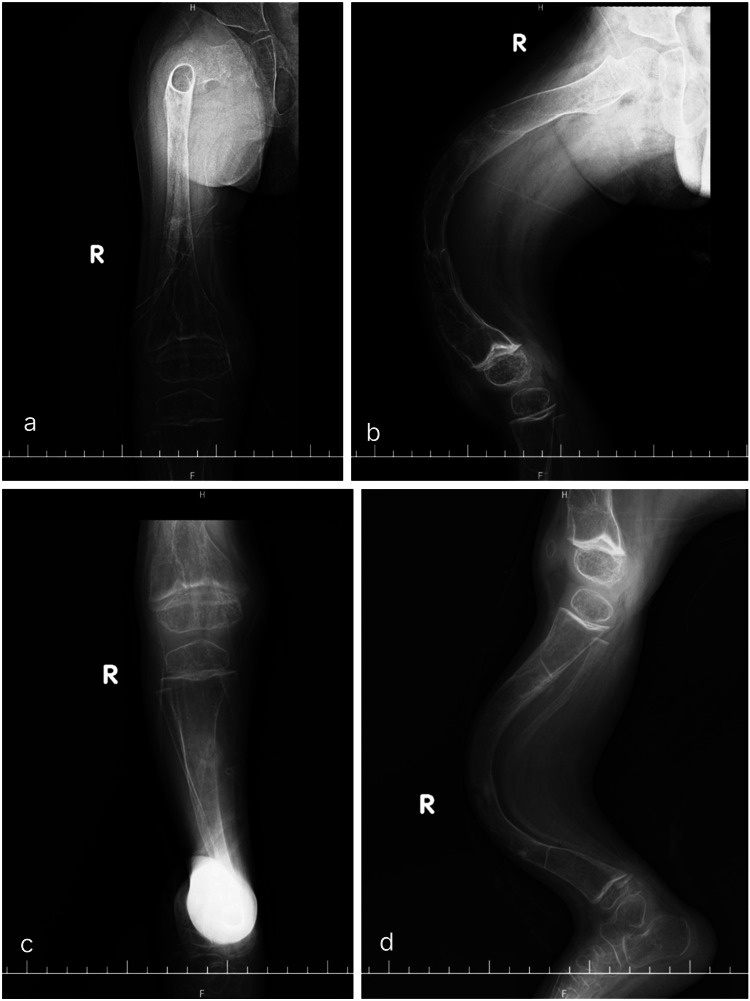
(a-d) Plain radiographs showing deformity of the right lower limb
